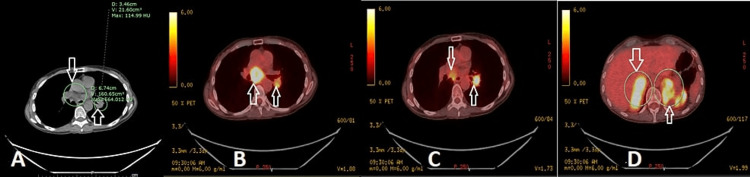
Positron emission tomography(PET)/Computed tomography (CT) Positron emission tomography (PET)/Computed tomography (CT) scan showing evidence of hypermetabolic left hilar mass (B and C) with metastasis to bilateral adrenal glands (D).
